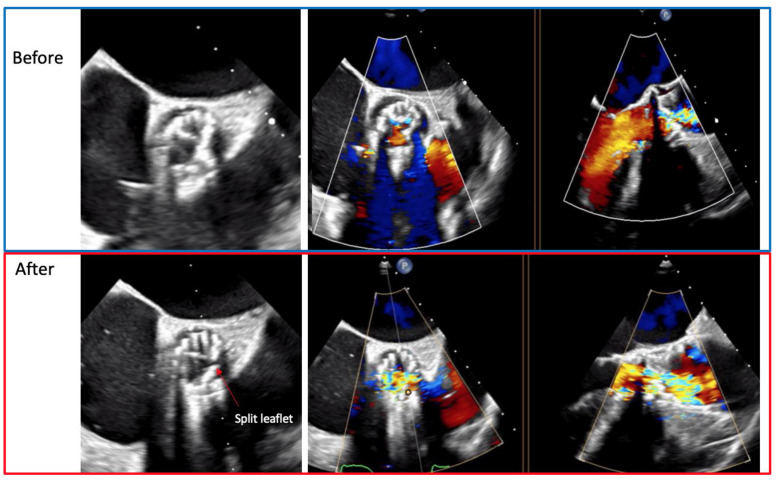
Transesophageal echocardiogram showing the left coronary leaflet before and after the laceration.
